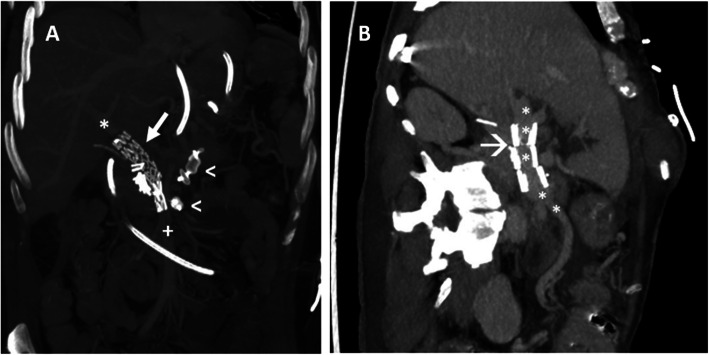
a Patient #1: Post-procedural CT scan showing a patent superior mesenteric vein (white cross) and portal vein (asterisk), with the stent-graft deployment (white arrow) and two vascular plugs (arrow-heads) at the splenic and inferior mesenteric vein origins. b Patient # 2: CT scan showing the correct deployment of the stent (arrow), with a patent portal vein (asterisks)
